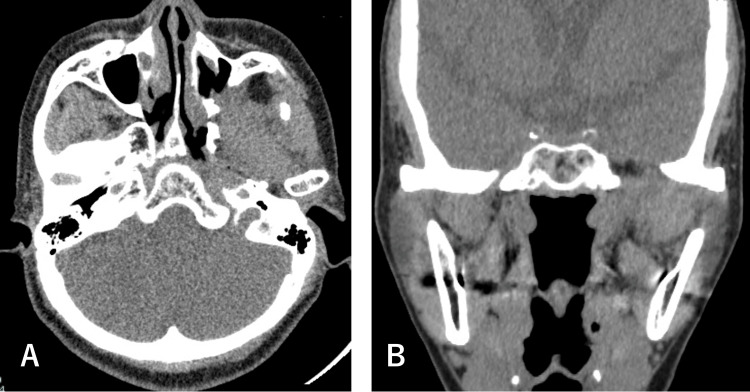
Postoperative CT scans of Case 2 at three-year follow-up. (A) Axial view and (B) coronal view demonstrate the effective management of the swelling, with no evidence of recurrence.
